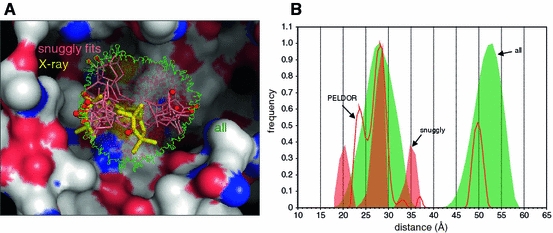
Example 4: The octameric outer membrane channel Wza. a All conformations found by mtsslWizard are shown in translucent and surrounded by a green outline to indicate the size of the whole distribution. Those conformations flagged as “snuggly fits” are shown as *red sticks*. The two conformations from the X-ray structure are colored *yellow*. The molecular surface of the protein is colored according to atom type. Carbon: *gray*, oxygen: *red*, nitrogen: *blue*. b The corresponding histograms for the 1–2 and 1–3 distances of Wza. The histograms for all conformations are filled *green*; the histograms for the “snuggly fit” conformations are colored *red*. Experimental PELDOR data were taken from [16]. Note that both the *red* and *green* histograms are individually normalized, otherwise the “snuggly fit” histogram would not be visible on the chosen *y*-axis scale
